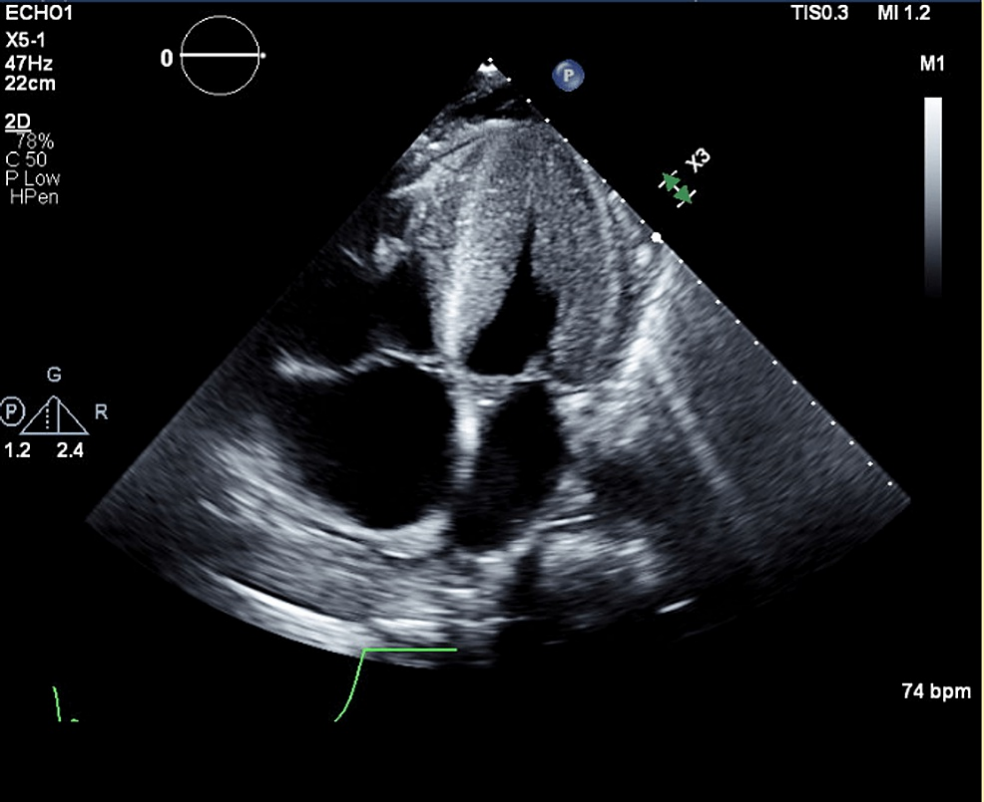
Apical 4 Chamber View - Transthoracic Echocardiogram
